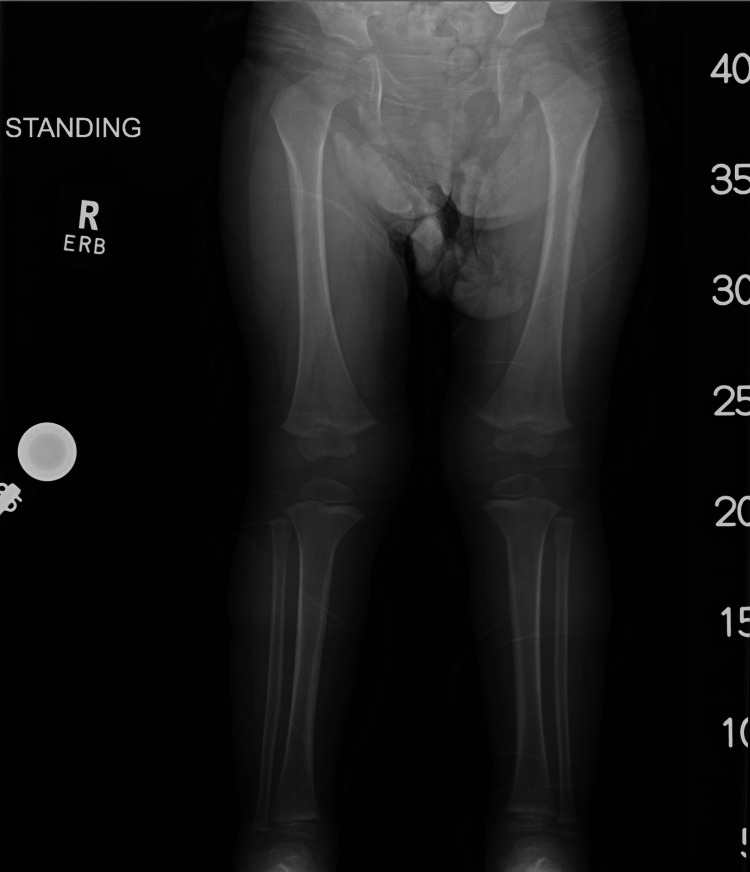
Standing anteroposterior hip-to-ankle radiographs of bilateral lower extremities at two years of age showing normal appearance of the long bones.
